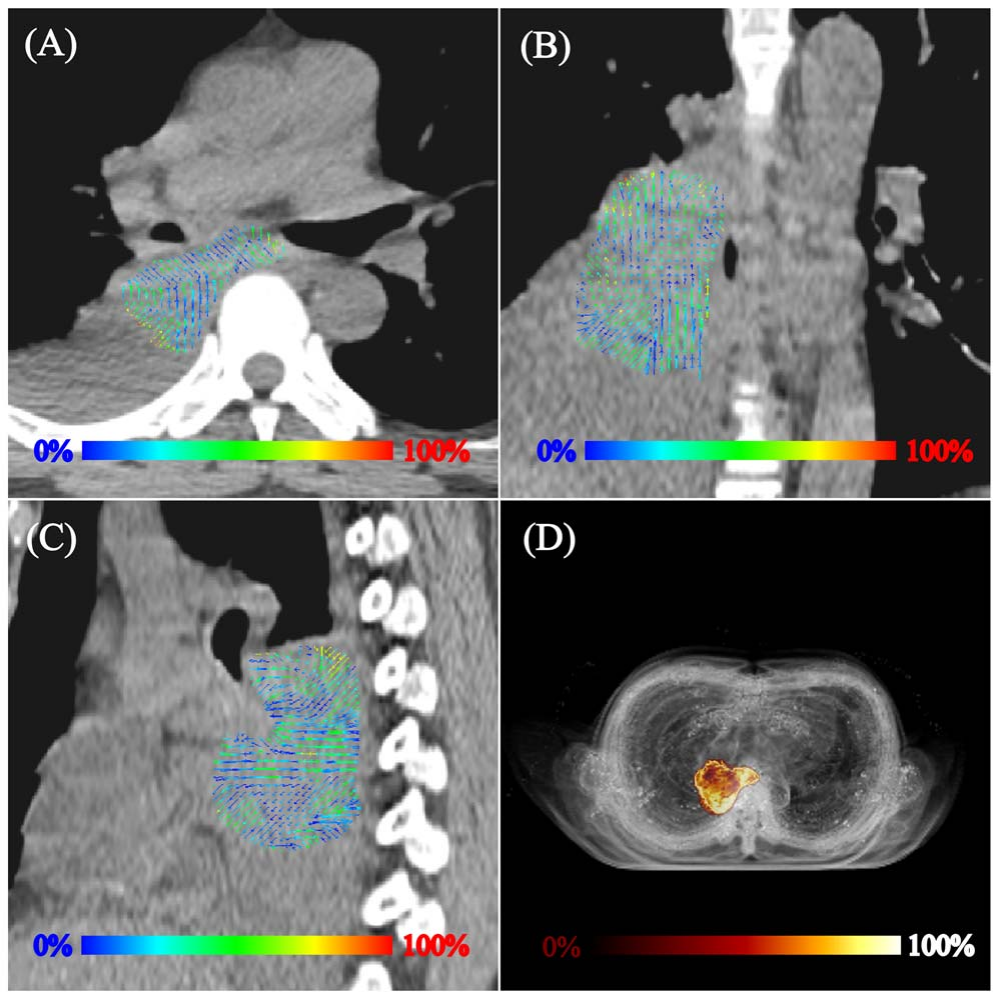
The projection of the tumor deformation vectors with the color scale superimposed on the CT scan for lung cancer patient 3 in the (A) transverse, (B) coronal and (C) sagittal views. (D) A rendering of the tumor deformation with a scout view of the thoracic CT for the same patient.
